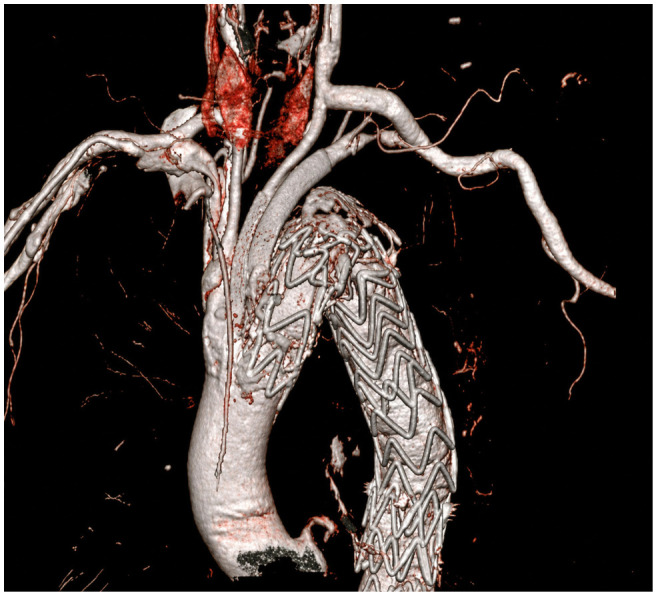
Three-dimensional computed tomography angiography demonstrating thoracic endovascular aortic repair with in situ fenestration and supra-aortic branch revascularization of the left subclavian artery.
